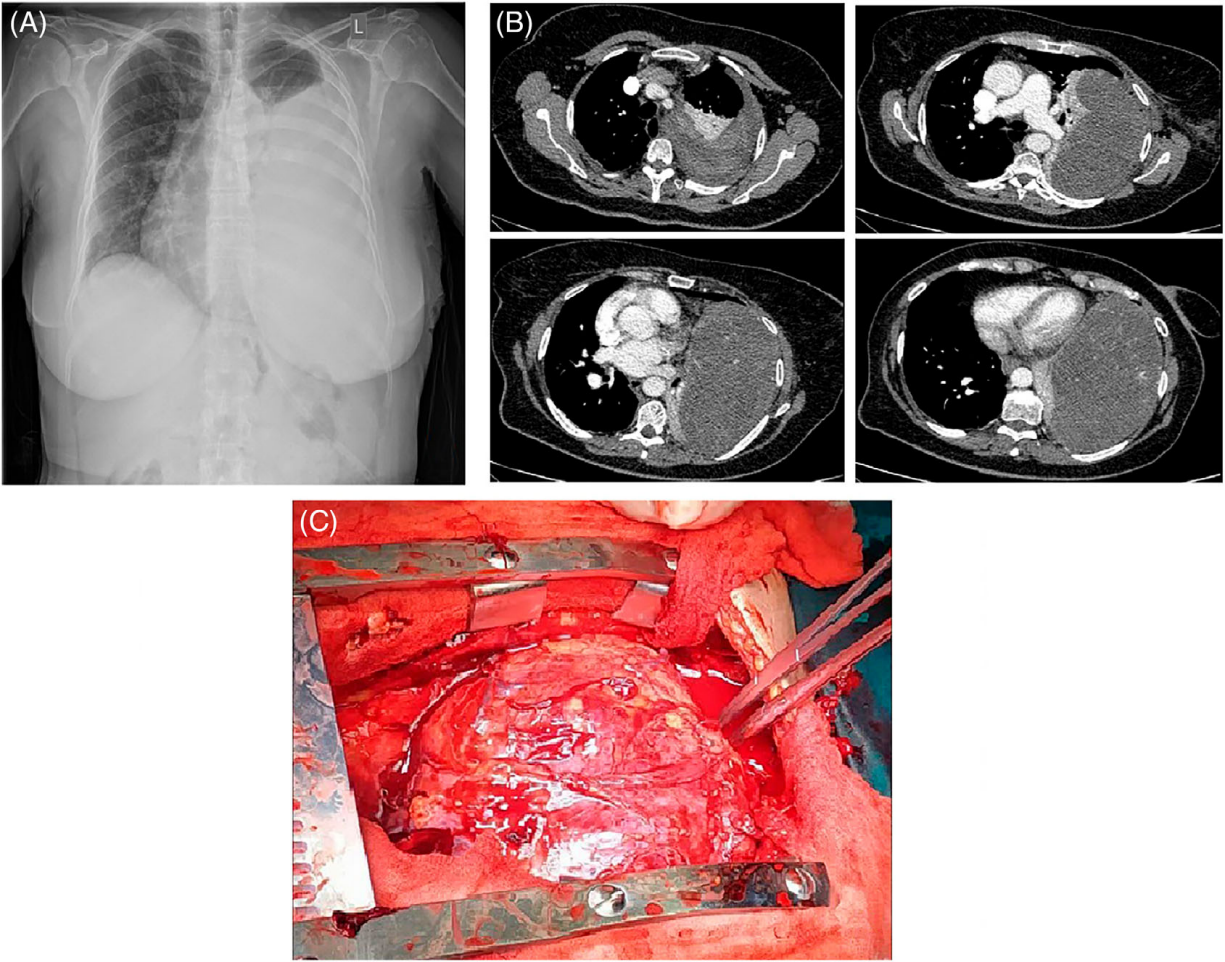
(A) Chest x‐ray revealing opacification of the left middle and lower pulmonary fields. (B) CT scan of the chest showing a well circumscribed, soft tissue mass occupying almost the entire left hemithorax, atelectasis and a small left pleural effusion. (C) Gross appearance of the resected tumour. Its dimensions were 19.2 × 18.6 × 8.6 cm and its weight was approximately 5 kg
